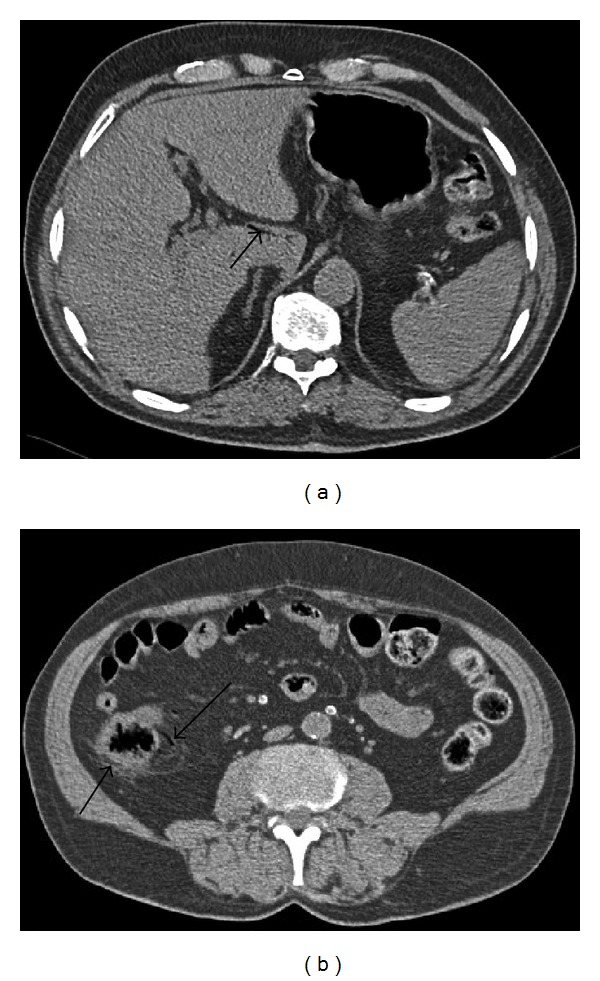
(a) Noncontrast CT image of the liver demonstrates intraluminal gas in a portal venule in the caudate lobe (arrow). (b) Noncontrast CT image through the lower abdomen demonstrates cecal wall thickening with fat stranding and linear pneumatosis intestinal within the cecal wall with intraluminal gas in adjacent mesenteric venules (arrows). Note the calcified mesenteric artery branches.
